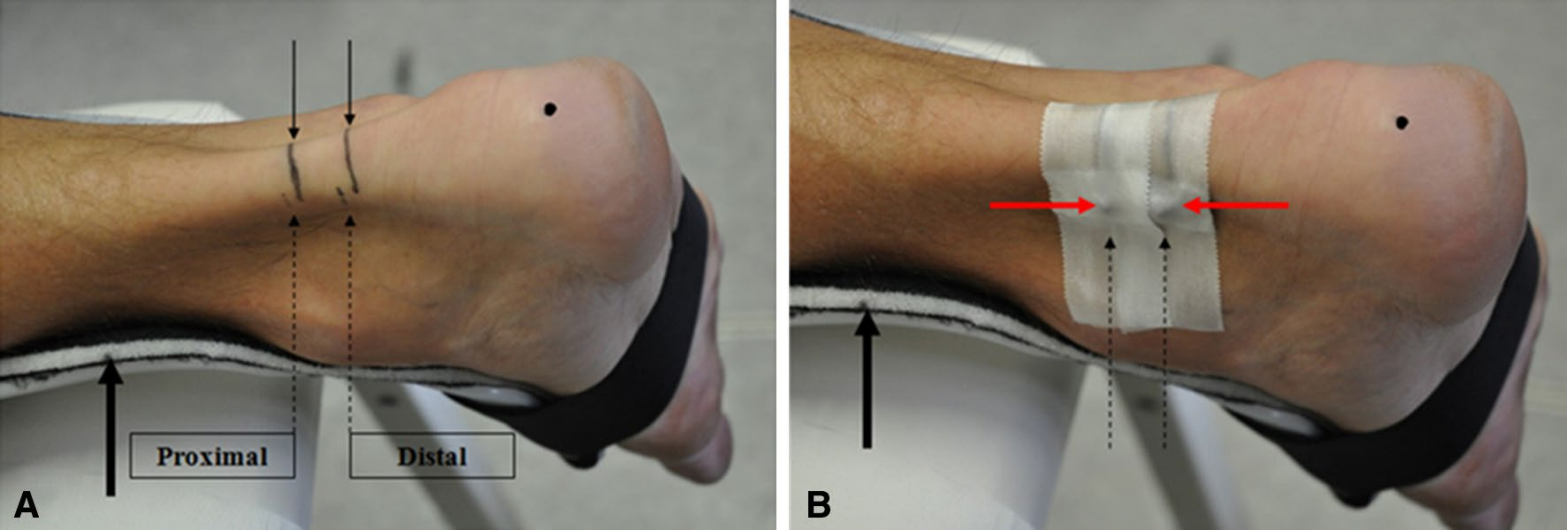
Subject’s ankle joint with the attached splint (*arrow* in *bold*) a initial preparation for measurements and b the ankle joint prepared for the MRI examination. *Black arrows* indicate measurement landmarks, whereby *dashed arrows* indicate the measurement positions (distal, proximal) defined with respect to the footprint of the transducer. *Red arrows* indicate the spherical markers attached to the measurement positions. The *black spot* on the heel indicates the tuberositas calcanei (color figure online)
